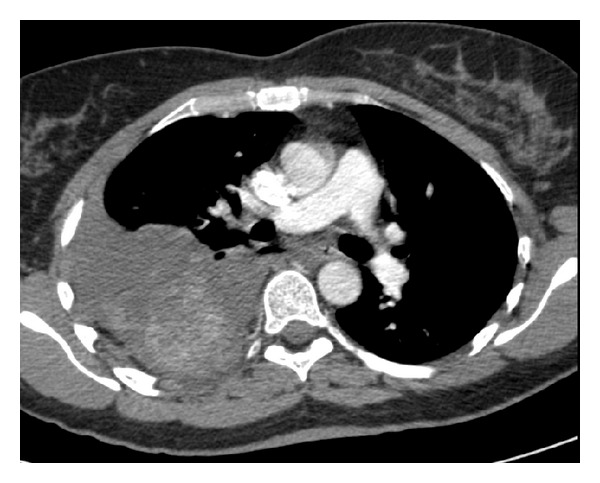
CT scan showing a right pleural effusion consistent with a haemothorax due to recent and active bleeding associated with a paravertebral hyperdense formation (7 cm in diameter) suspected to be a parietal neoplasm.
